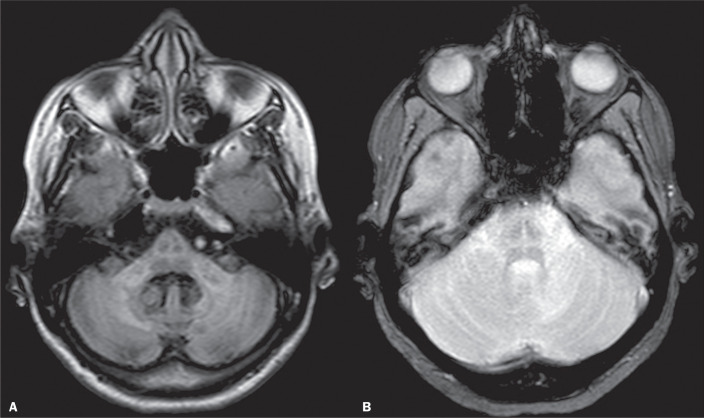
Axial fluid-attenuated inversion recovery sequence (A) and axial T2-weighted image (B) showing a cruciform signal (“hot cross bun sign”) involving the transverse fibers, the median raphe of the pons and the middle cerebellar peduncles, findings that are consistent with JC virus infection.
